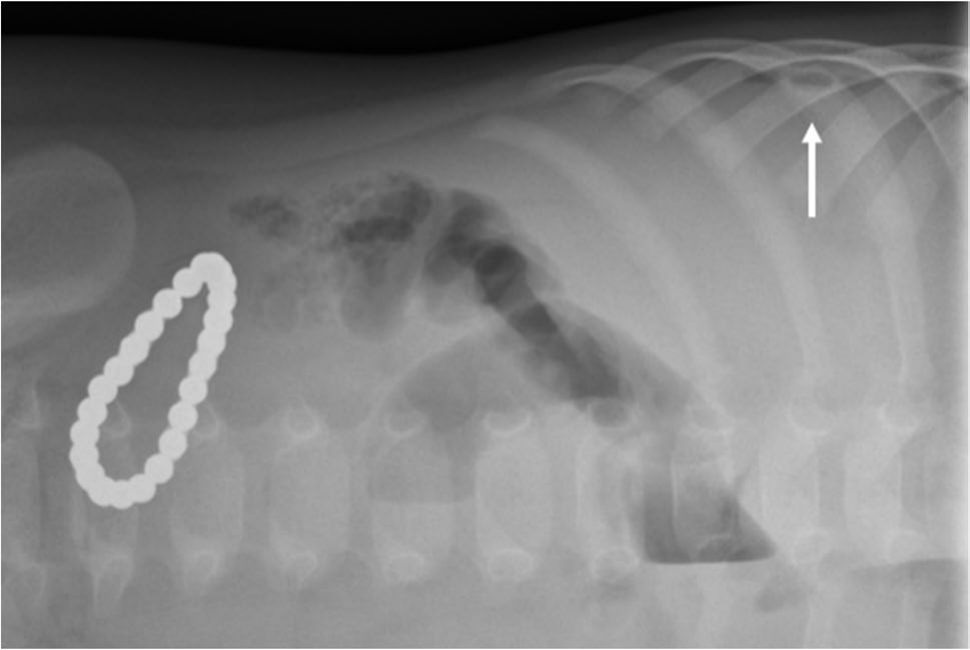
Magnification of the right decubitus view radiograph (1-year-old boy) shows a small quantity of free air between the liver and the diaphragm (*arrow*). Surgery verified perforation of four small bowel loops and 25 small magnetic balls
